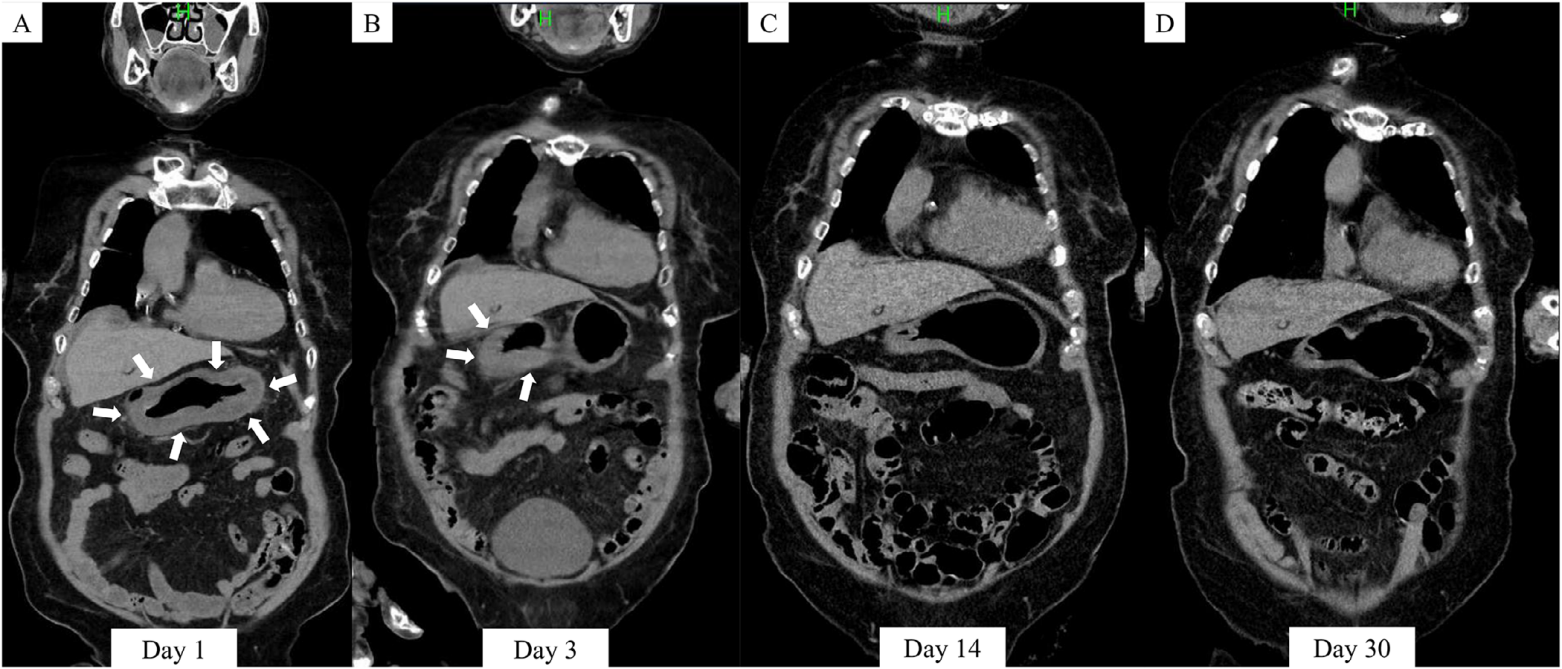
Computed tomography (CT) findings. (A) Abdominal CT revealed circumferential edematous thickening of the gastric wall. No evidence of pneumomediastinum or free air in the abdominal cavity was observed. (B–D) Follow‐up CT demonstrated gradual improvement in the circumferential gastric wall thickening.
